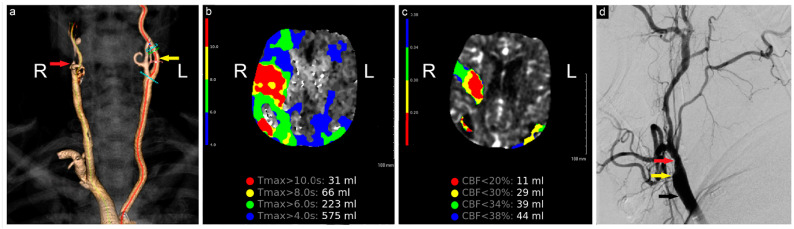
Preoperative neuroimaging. (a) CTA shows complete occlusion of the right ICA; (b) computed tomography perfusion study shows a large region of T_max_ > 6 s (time to maximum of the tissue residue function) measuring 223 mL, primarily involving the right middle cerebral artery territory, (c) with a smaller region of CBF< 30% measuring 29 mL in the same territory. The mismatch volume and ratio were 194 mL and 7.7, respectively, with a hypoperfusion index of 0.1; (d) angiogram shows complete occlusion of the right ICA (yellow arrow), patent ECA (red arrow), and CCA (black arrow). R: Right, L: Left, CBF: Cerebral Blood Flow.
